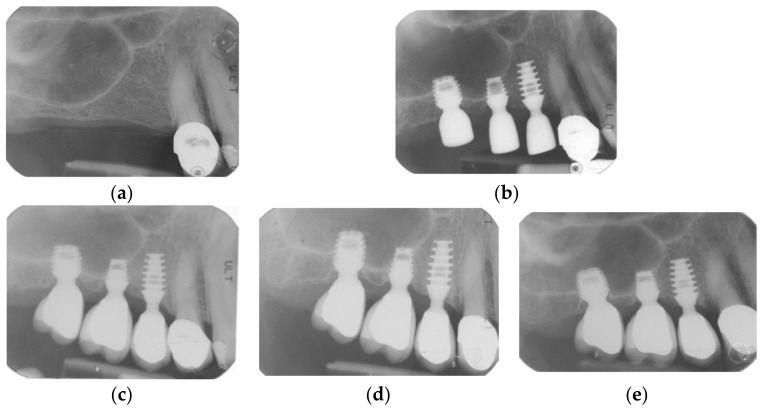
Single implants placed in 1.5, 1.6, and 1.7 sites (4 × 6 mm, 4 × 5 mm and 4.5 × 5 mm): (a) pre-operative radiograph before implant placement; see minimal bone levels (1.5 RCBH = 5.65 mm; 1.6 RCBH 4.2 mm; 1.7 RCBH = 3.5 mm); (b) Radiograph obtained at time of placement; see augmented sinus floor (1.5 sinus lift = 2 mm; 1.6 sinus lift = 2.2 mm; 1.7 sinus lift = 2 mm); (c) radiograph obtained at time of loading; see clear protrusion of two implants into the sinus; (d) radiograph obtained at 3-year follow-up; (e) radiograph obtained at 5-year follow-up. See large graft resorption after 3 years: the material immediately placed can be seen only with great magnification; it is then completely resorbed; however, it can be observed that finally the new necessary functional bone has been formed. However, see stable bone levels with minimal changes between 3-year (1.5 RCBH = 5.8 mm; 1.5 sinus lift = 2 mm; 1.6 RCBH = 4.2 mm; 1.6 sinus lift = 2 mm; 1.7 RCBH = 2.7 mm; 1.7 sinus lift = 0.5 mm) and 5-year follow-up (1.5 RCBH = 5.5 mm; 1.5 sinus lift = 1.8 mm; 1.6 RCBH = 3.8 mm; 1.6 sinus lift = 1.5 mm; 1.7 RCBH = 2.5 mm; 1.7 sinus lift = 0.5 mm).
